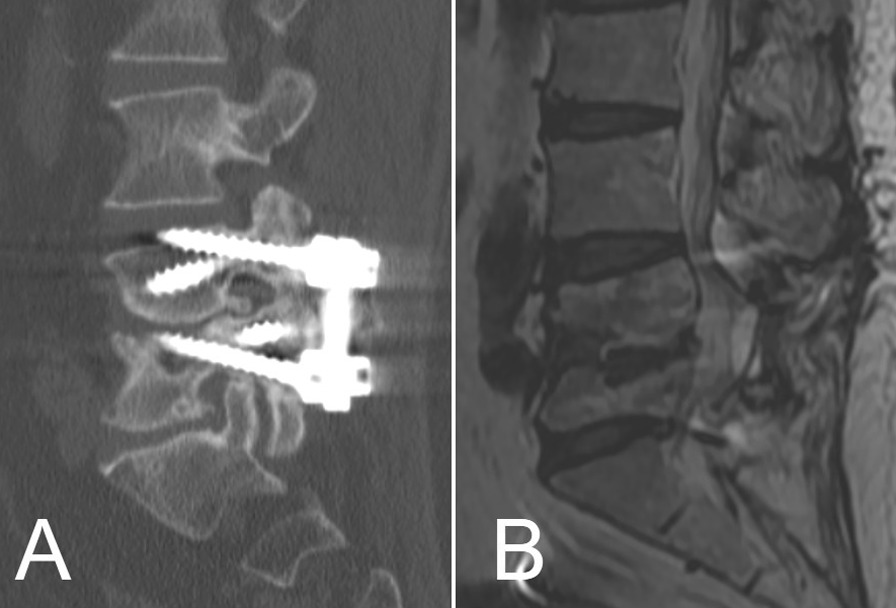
The CT (A) and MRI (B) at 2.5-year follow-up. CT showed ultimate bony fusion of the L4–5 segments and penetration of a CBT screw into the L3–4 interbody space. MRI showed no recurrence but adjacent segmental degeneration and asymptomatic canal stenosis
